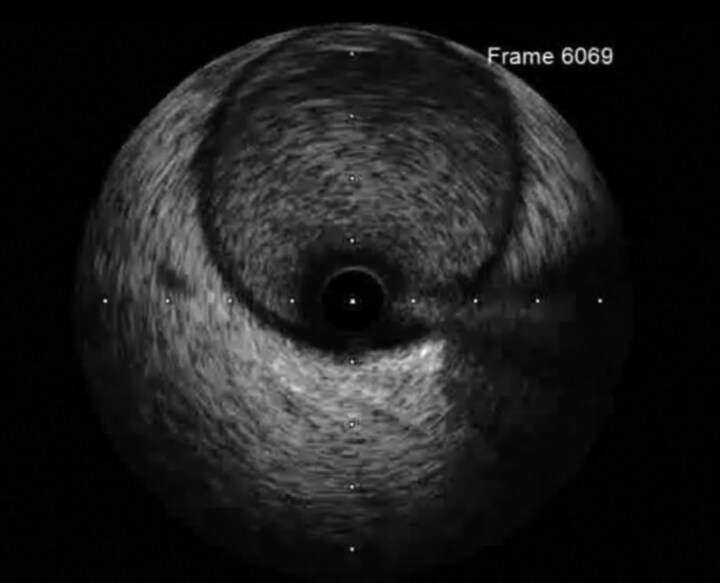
Intravascular ultrasound. A large, homogeneous intraluminal thrombus without calcification or atherosclerotic plaque. The vessel wall appears smooth and intact, inconsistent with plaque rupture or erosion.
